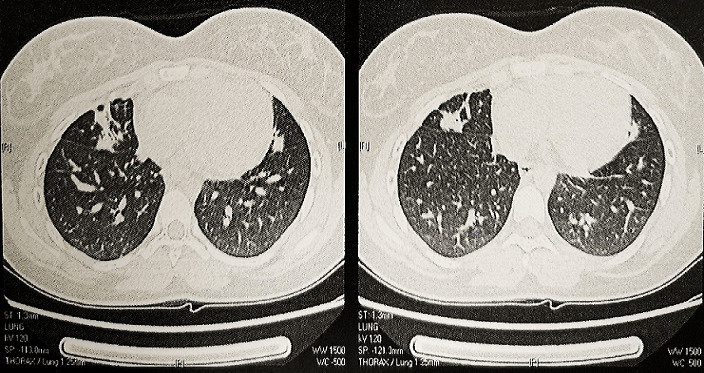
Chest CT scan in axial sections showing bilateral multiple nodules with ground-glass opacities (patient 2)
